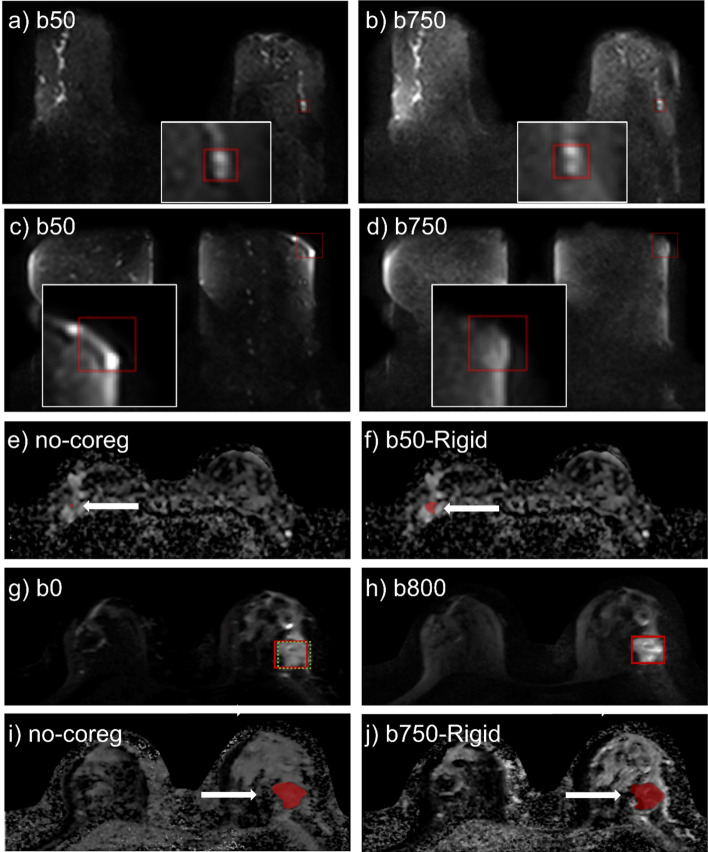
a and b show an example with slight misalignment of the lesion between the b50 and b750 images, with the lesion shifting a few pixels upwards in the b750 image. c and d demonstrate a case with misalignment or distortion between b50 and b750 images at the breast borders, not affecting the lesion itself. e and f show an example, where the same reader created different looking segmentations (red) on the ADC maps of two different methods. e ADC Map generated using non-co-registered b-values (no-coreg). f ADC Map generated using b50-Rigid co-registration method. g–j show an example, where there is a misalignment of the lesions visible in b0 (DWI with b-value of 0 s/mm^2^) and b800 (DWI with b-value of 800 s/mm^2^), yet the resulting lesion segmentations done by the same reader on both ADC maps remain similar. g shows the b0 DWI with a dotted green box showing the position of the lesion in b0 and a red box indicating its location in b800. h shows the b800 image with a red box indicating the lesion position. i ADC map generated using non-co-registered *b*-values (no-coreg). j ADC map generated using b750-Rigid co-registration method
